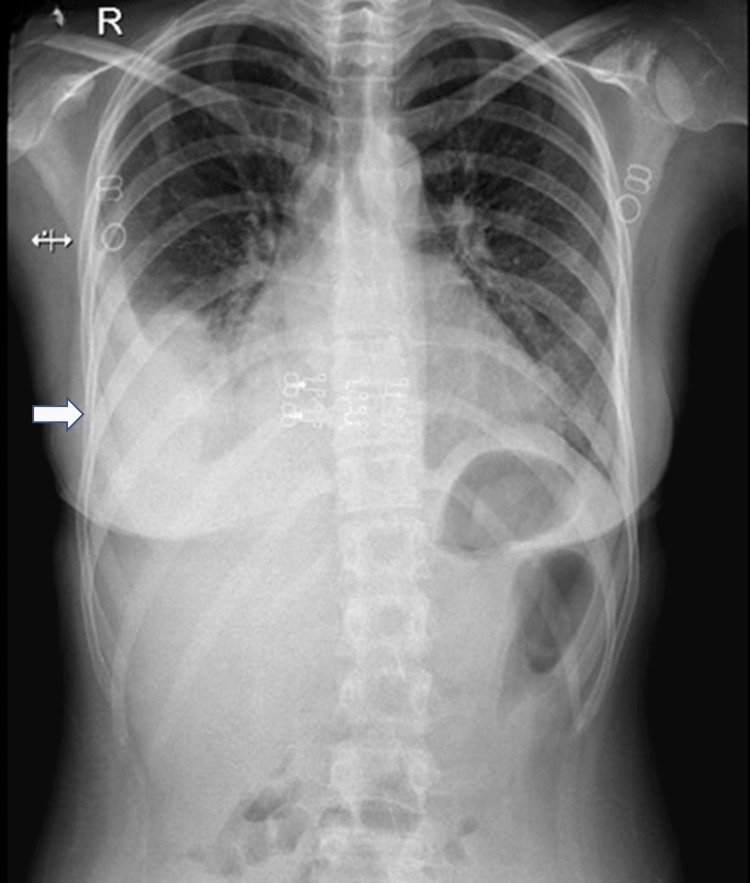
Chest radiograph (PA view) at presentation Chest X-ray (PA view) demonstrating right-sided pleural effusion. Homogeneous opacification of the right hemithorax with blunting of the costophrenic angle, consistent with a moderate-to-large pleural effusion at presentation. PA: posteroanterior
